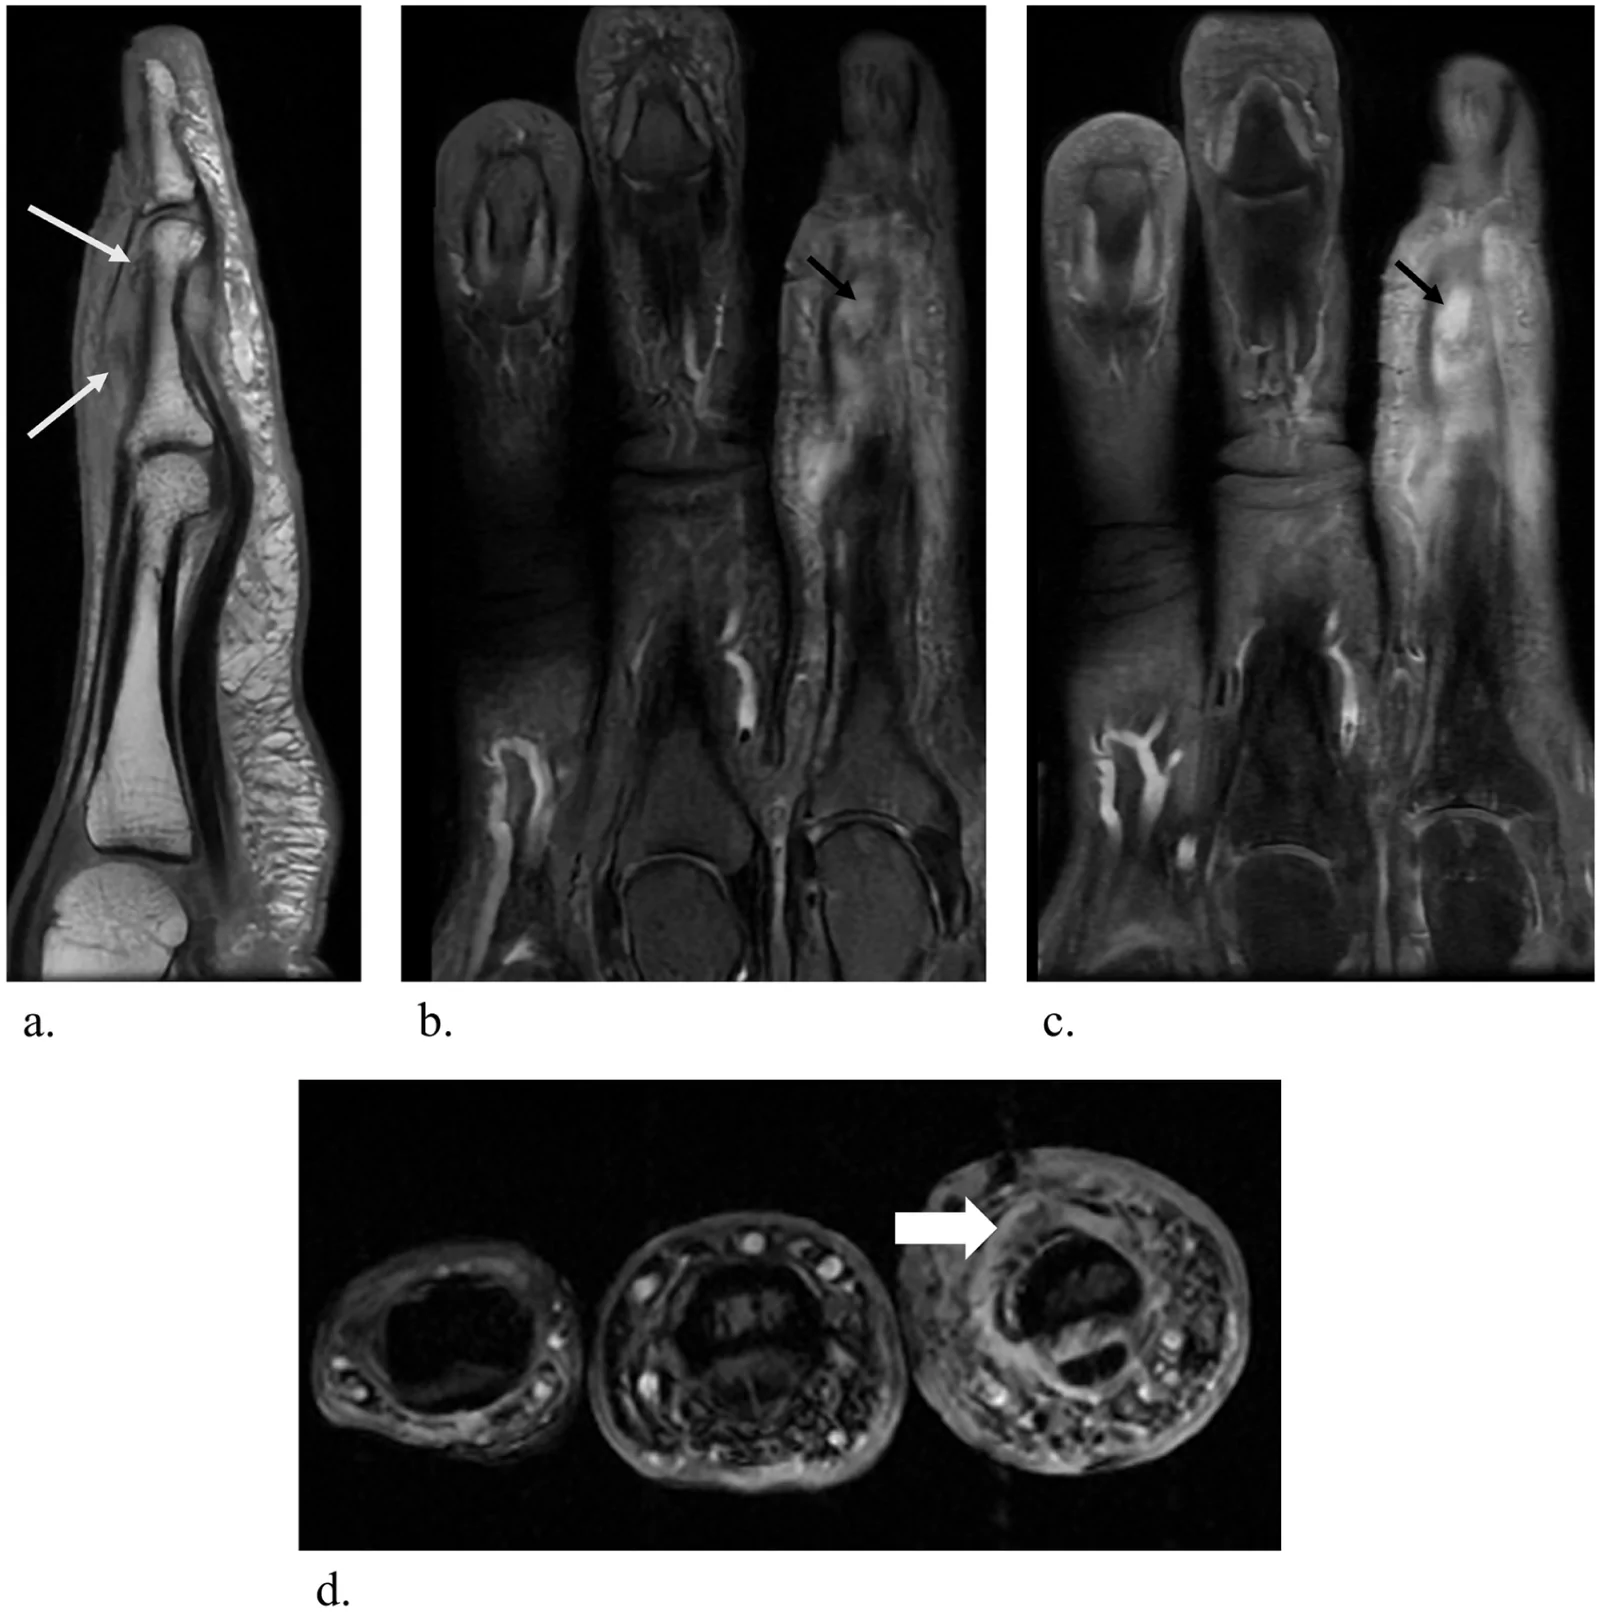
Magnetic resonance imaging performed 4 weeks post initial excision: additional sequences, including T2* and diffusion-weighted imaging, were performed due to suspicion of recurrence of fibro-osseous pseudotumour of the digit (FOPD). (A) Sagittal T1, (B) coronal T2 FS, (C) coronal T1 FS post-gadolinium, and (D) axial T2*. Nodular focal T2 hyperintense/enhancing solid lesion (black arrows) overlies the dorsal aspect of the shaft/neck of the second middle phalanx, compatible with FOPD recurrence. Sagittal T1 (A) and axial T2* (D) sequences confirm low T1 signal from mineralisation (thin white arrows) and susceptibility artefact (thick white arrow) overlying the dorsal cortex.
